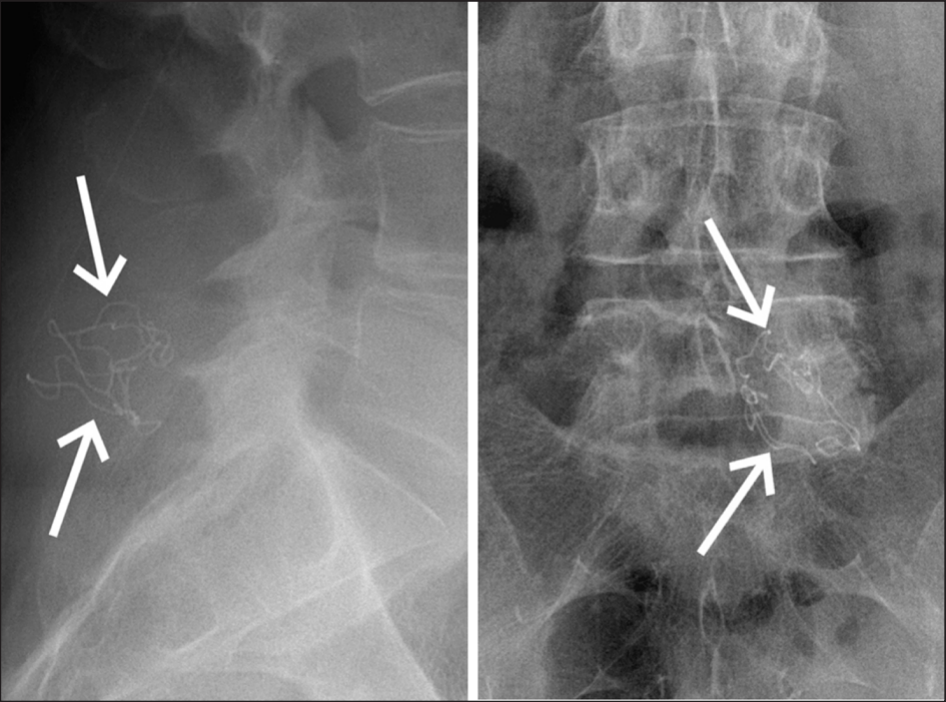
Lateral and frontal radiograph showing the contorted barium sulfate filament confirming the presence of a surgical gauze in the paraspinal soft tissues.
